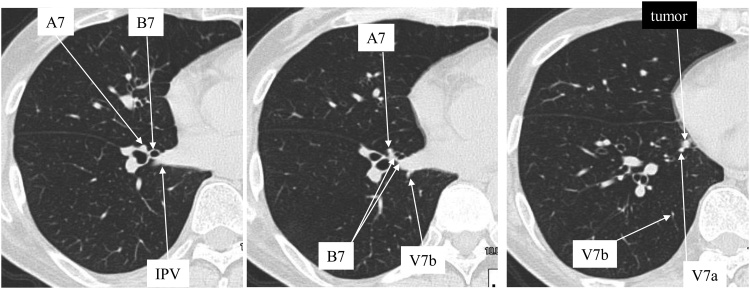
Preoperative computed tomography. A7 and B7 branch from the basal artery and basal bronchus, respectively, to run on the ventral side of the IPV. Therefore, the entire S7 is located ventral to the IPV. A 6-mm nodule can be seen in S7 adjacent to V7a. A thin V7b drains into V8-10.
